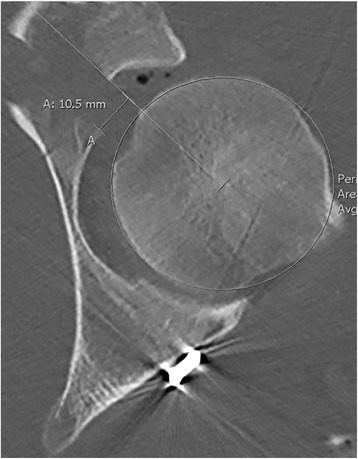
Example of postoperative CT scan (axial plane) with measurement lines for gaps
